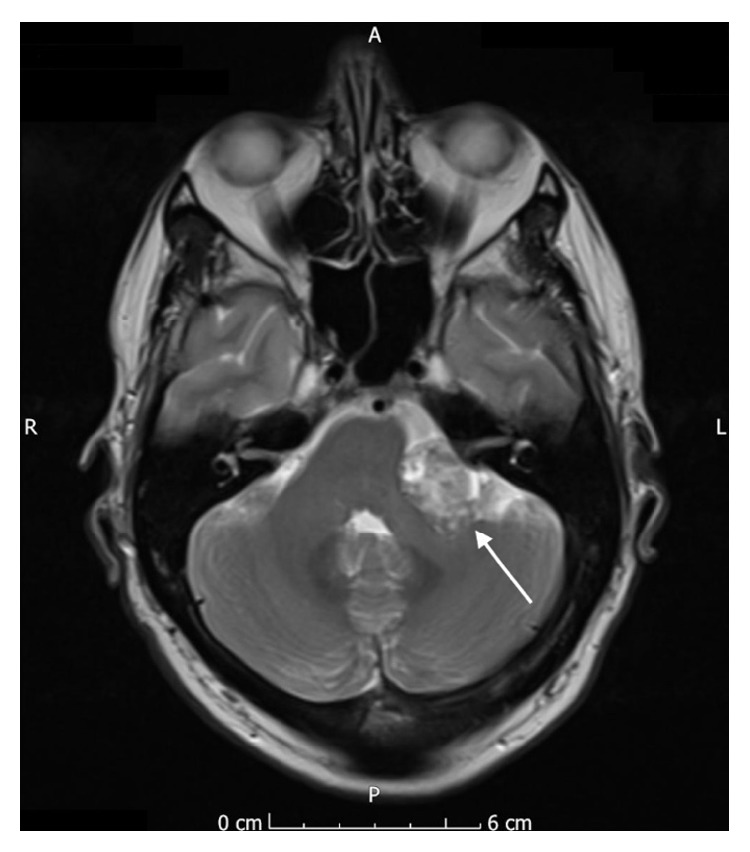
Axial MR image (T2 weighted, fat saturated) showing the vestibular schwannoma affecting the left vestibulocochlear nerve.
